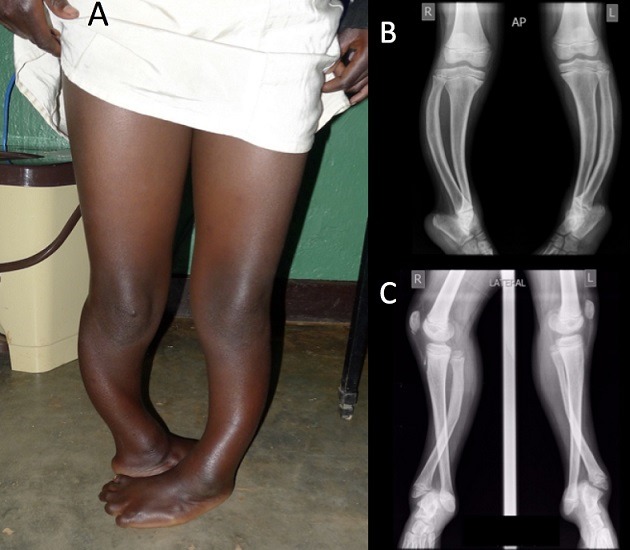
(A) bilateral marked endotorsion of the lower limb with 90° internal rotation of the foot; (B) X-ray evaluation AP view; (C) X-ray evaluation lateral view
